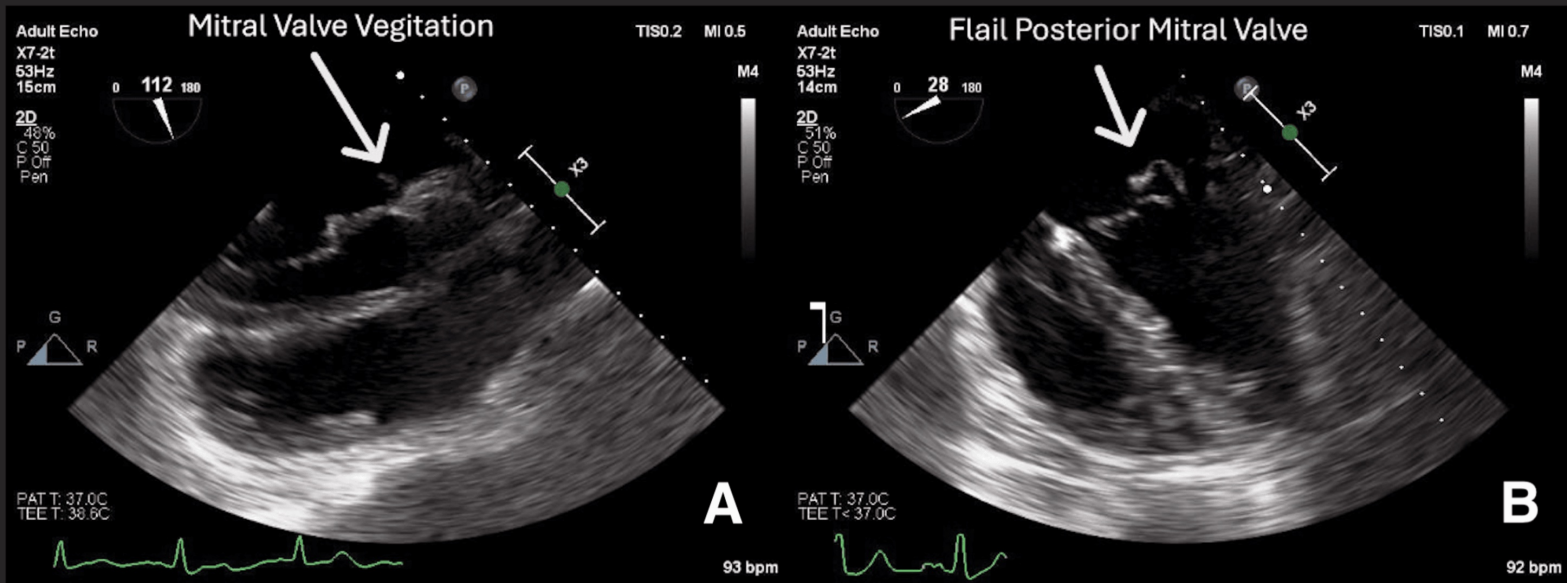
Transesophageal echocardiogram (TEE) demonstrated visible mobile vegetations attached to the mitral leaflet (A); TEE revealed a flail posterior mitral valve leaflet (B).
